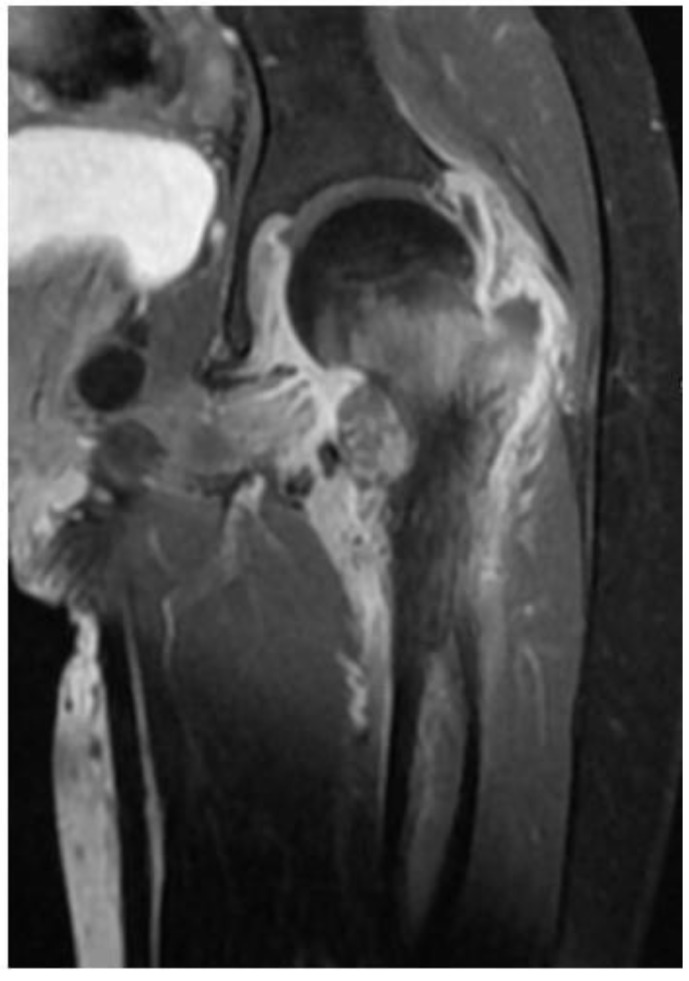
Magnetic Resonance Imaging (MRI) of the hip revealed bony overgrowth of the lesser trochanter with early cystic formation and a fluid-fluid level, extensive bone marrow edema, extensive adjacent soft tissue edema and hip joint effusion with evidence of synovial thickening and enhancement post contrast administration.
